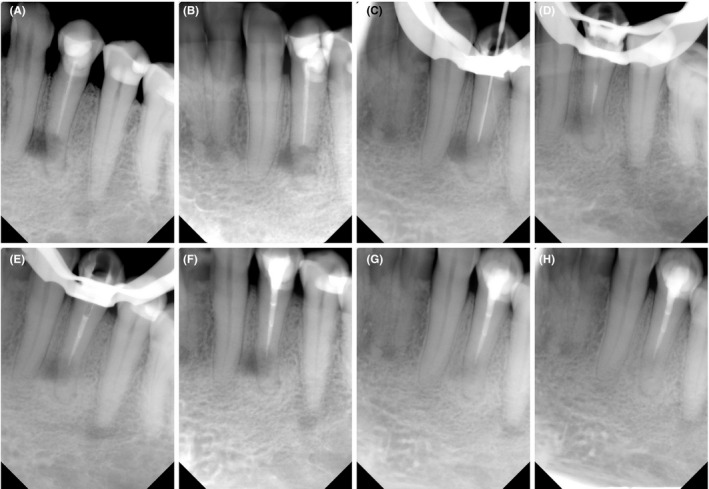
A, Preoperative periapical radiograph (straight angulation), B, preoperative radiograph (mesial angulation), C, working length radiograph, D, MTA apical plug radiograph, E, postobturation evaluation radiograph, F, postoperative periapical radiograph, G, 1‐y follow‐up radiograph, and H, 2‐y follow‐up radiograph
